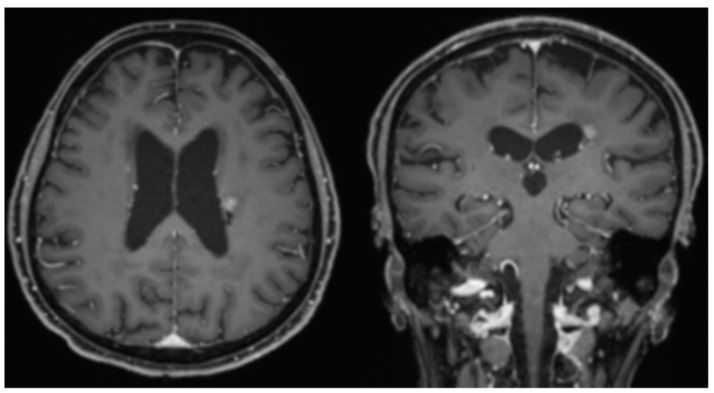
Brain MRI after 6 cycles of reduced-dose HD-MTX induction therapy, showing significant response of lymphoma to the treatment on post-contrast T1 images.
